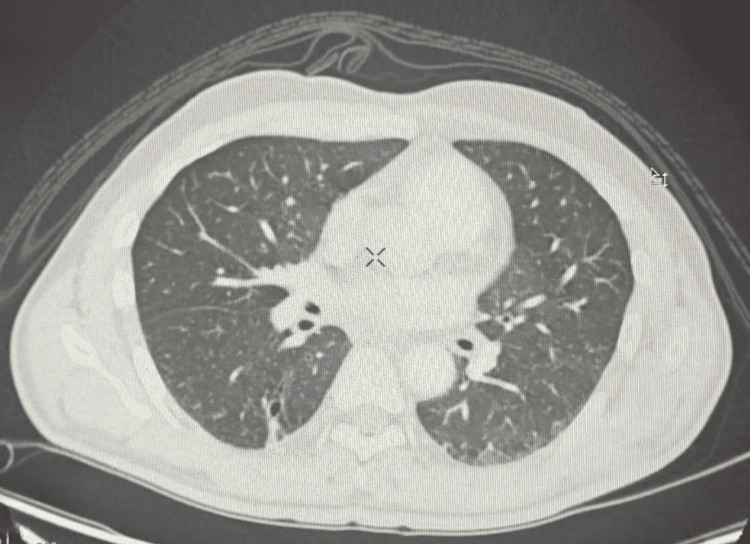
CT chest demonstrated the right lower lobe lesion, appearing as air cyst/thin-walled cavity measuring 5 x 7 x 13 mm surrounding with linear atelectatic bands
